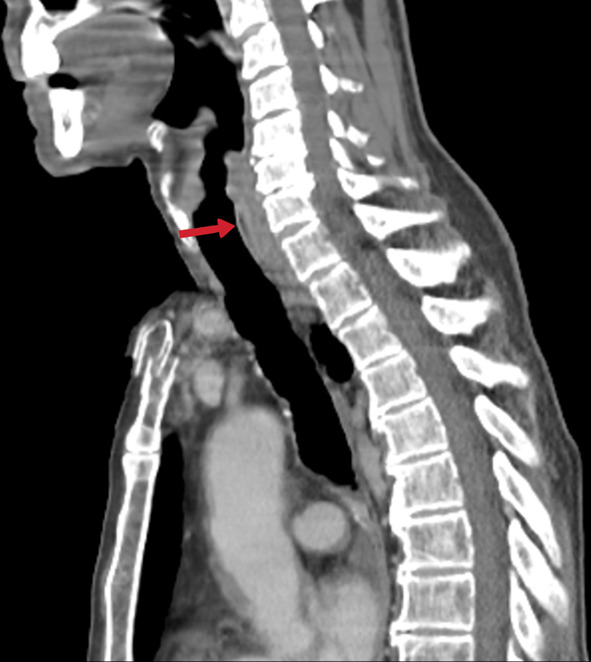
A 68-year-old-male. Sagittal reformatted contrast-enhanced CT image reveals an increased thickness of the larynx's posterior wall (arrow). Sternum fracture is also noted in the manubrium
